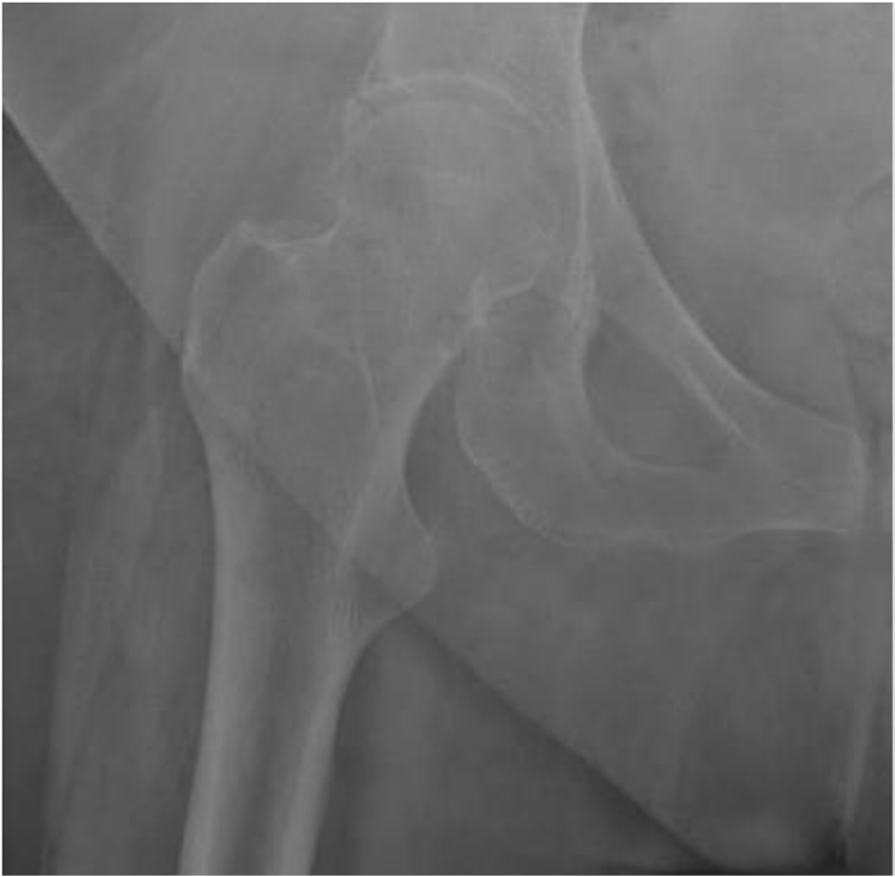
Example of blinded radiographs included in the study. (1) Femural neck fracture with high intra and inter observer reliability. (2) Femural neck fracture with low intra and inter observer reliability
